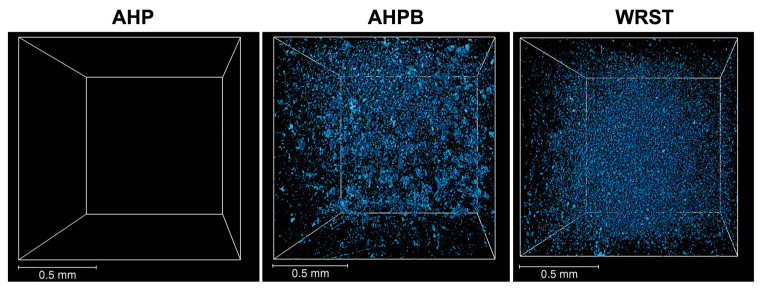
Volume rendering of the segmented pores (blue color) in: AH plus (AHP), AH Plus Bioceramic (AHPB) and Well-Root ST (WRST) obtained by X-ray tomography analysis. The scale bar corresponds to 0.5 mm in all images.
